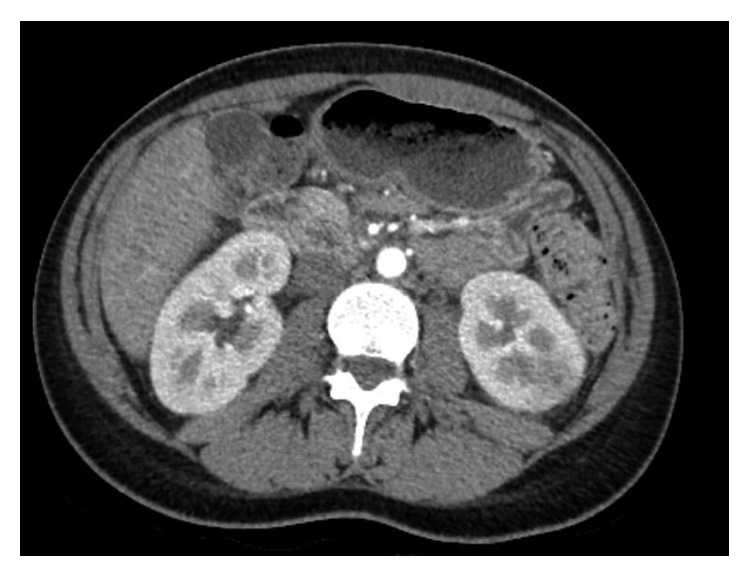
Contrast-enhanced computed tomography demonstrating a hypoenhancing region in the left kidney.
